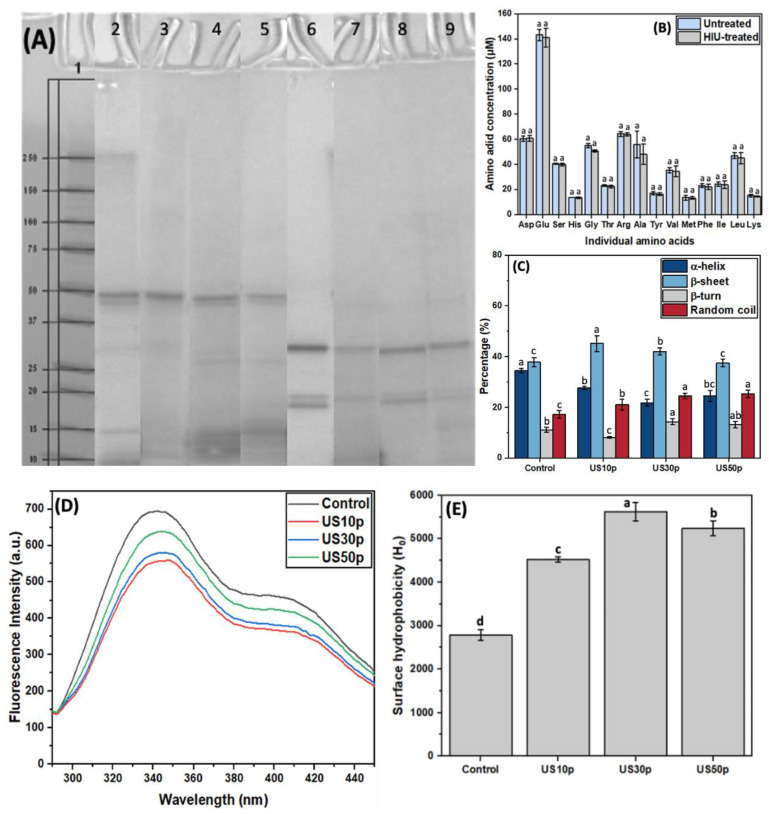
(A) SDS-PAGE profiles of control HPI and US-treated HPI with (reducing) or without (non-reducing) the addition of β-ME. Lanes in SDS-PAGE profiles: Lane 1: Marker; Lane 2: Conventionally extracted HPI; Lane 3: 11.2 W/cm^2^, 5 min US; Lane 4: 26.8 W/cm^2^, 5 min US; Lane 5: 50.0 W/cm^2^, 5 min US. Lanes 2–5 are under non-reducing conditions, whereas lanes 6–9 are their corresponding reducing SDS-PAGE profiles; (B) The amino acid profiles of untreated HPI and HIU-treated HPI; (C) The secondary structure of untreated and US-treated HPI under different power densities (11.2, 26.8, and 50.0 W/cm^2^ for 5 min); (D) Fluorescence spectra indicating tertiary structural differences between conventionally extracted and US-treated HPI under different power densities (11.2, 26.8, and 50.0 W/cm^2^ for 5 min); (E) Changes in surface hydrophobicity (H_0_) of conventionally and high-intensity ultrasound extracted (11.2, 26.8, and 50.0 W/cm^2^ for 5 min) HPI. The experiments were conducted in triplicate. Different lowercase letters in (B,C,E) represent the significant differences among different US treatments (*p* < 0.05).
